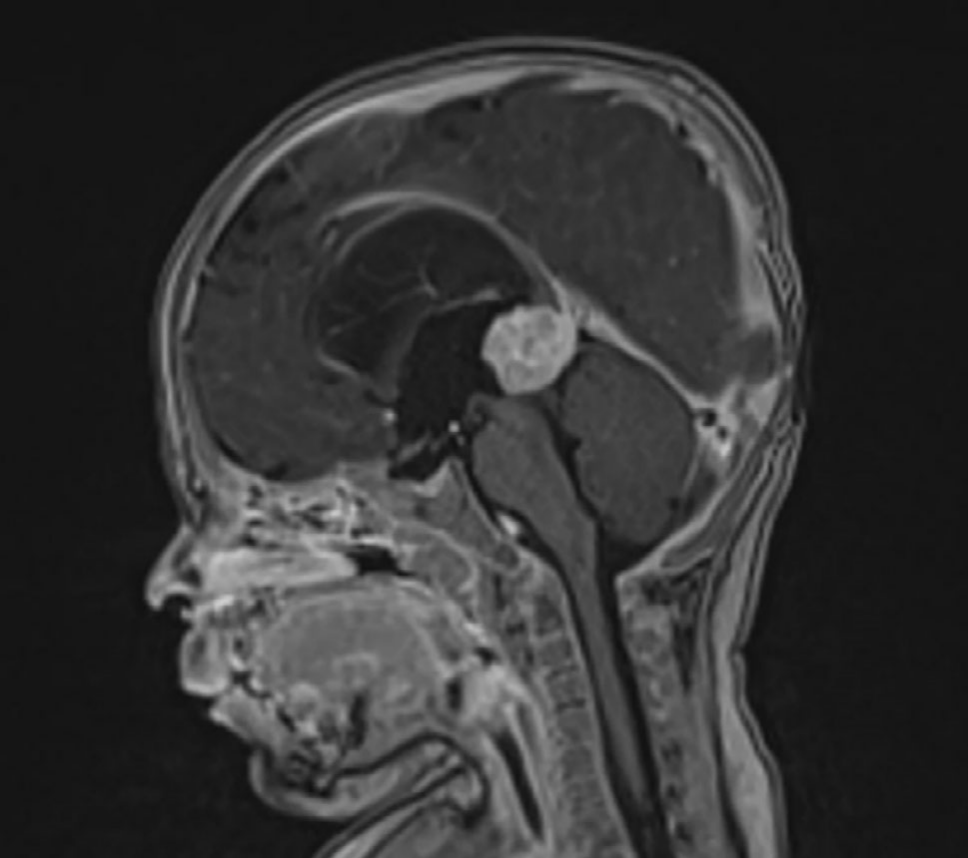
MRI, sagittal plane, T1 sequence after gadolinium injection.
